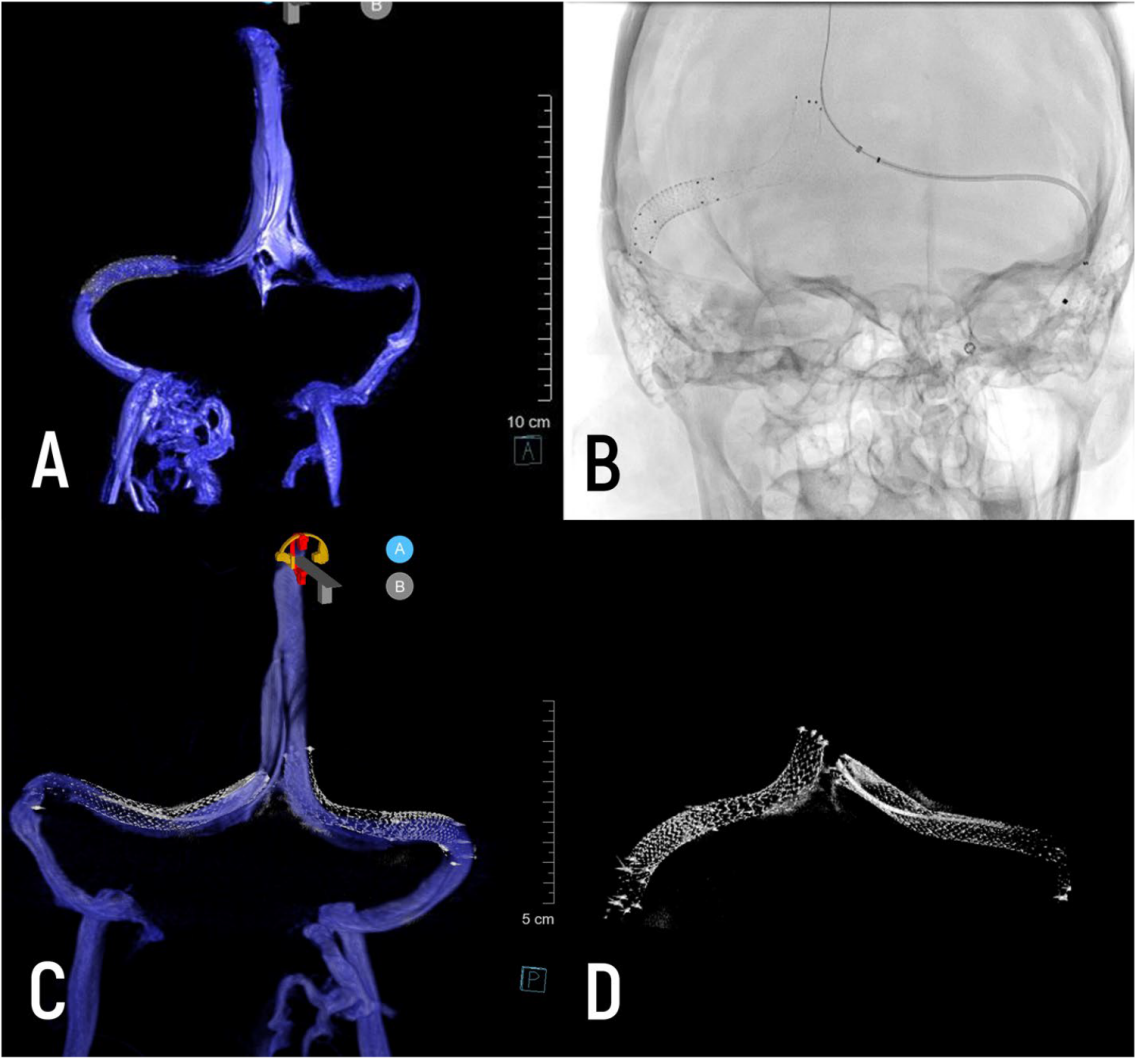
(A) Diagnostic 3D-RV after right transverse sinus stenting; (B) silver stenting system advanced to superior sagittal sinus for left transverse sigmoid stenting; (C, D) bilateral stenting of both transverse sinuses, visualized with 3D-RV and micro-dyn CT.
